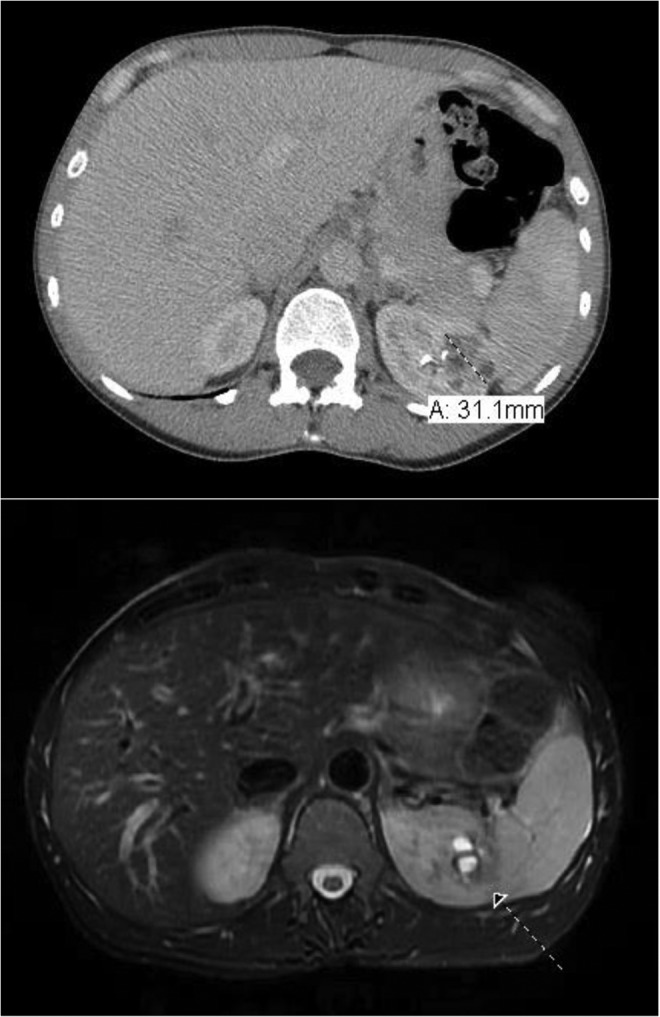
(*Top*) Axial CT image capturing the 3.1 cm *left upper pole* renal mass. (*Bottom*) Axial MRI T2 sequence demonstrating cystic components to the renal lesion. *Arrow* indicates the renal mass.
